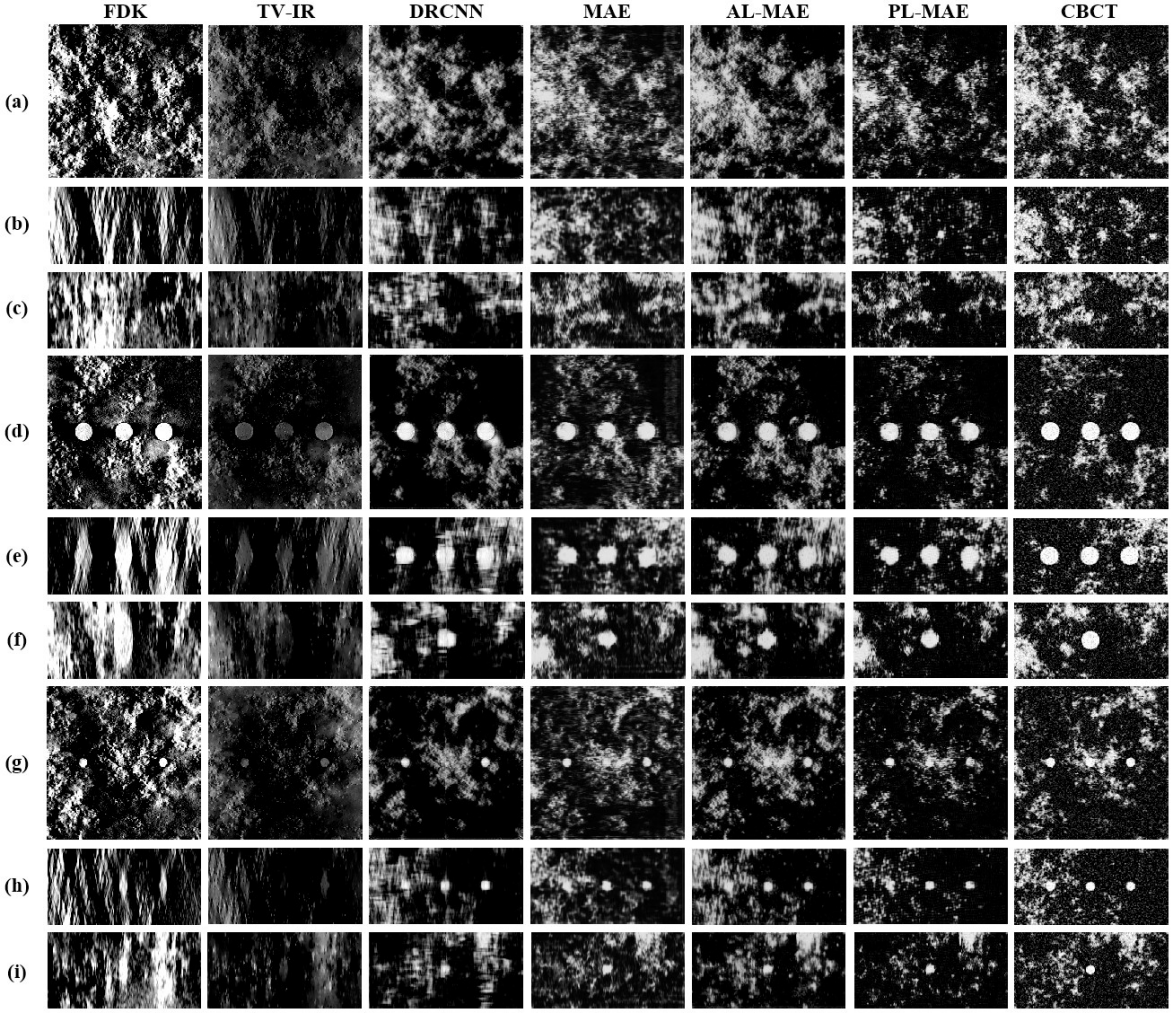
Results using 15% VGF DBT images. VGF 15% DBT images reconstructed with FDK and TV-IR, deblurred images by DRCNN, deblurred images by the proposed method with MAE, AL-MAE, and PL-MAE loss functions, and CBCT images (from left to right). Images without mass lesion for (a) axial, (b) coronal, and (c) sagittal planes; images containing 4 *mm* lesions for (d) axial, (e) coronal, and (f) sagittal planes, and images containing 2 *mm* lesions for (g) axial, (h) coronal, and (i) sagittal planes. The display window is [0.0456 0.0844] in *cm*^−1^.
